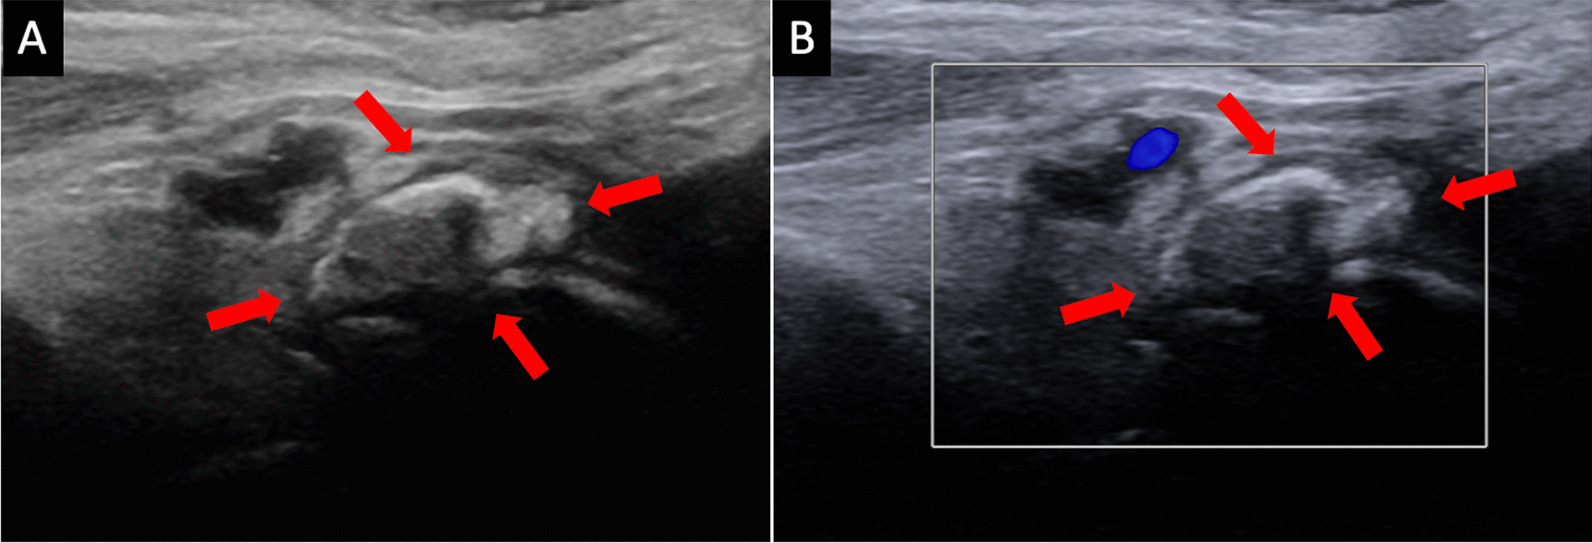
(A) Longitudinal grayscale and (B) color Doppler ultrasound images of the ankle demonstrate hyperechoic foci with acoustic posterior shadowing, internal calcific content, and no appreciable internal vascularity (arrows)
